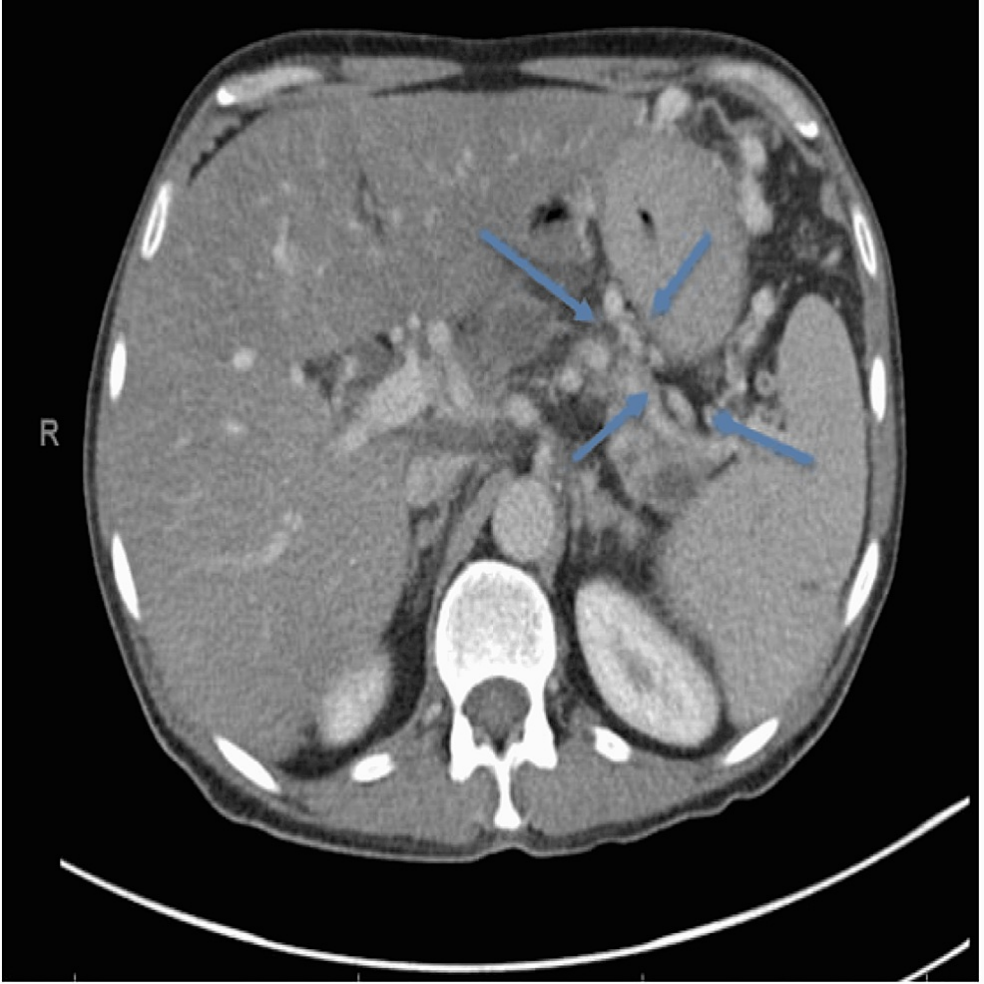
Computed tomography pulmonary angiogram (CTPA) with gastric varices (blue arrows).
